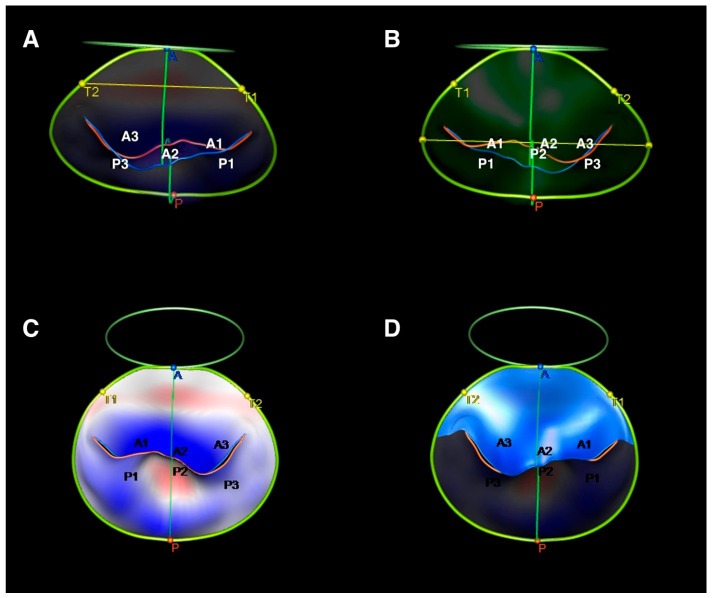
3D echocardiographic reconstruction of the mitral valve using Tomtec. (A) Measurement of the intertrigonal distance; (B) measurement of the commissural width; (C) measurement of the annulus area; (D) measurement of the anterior leaflet area.
